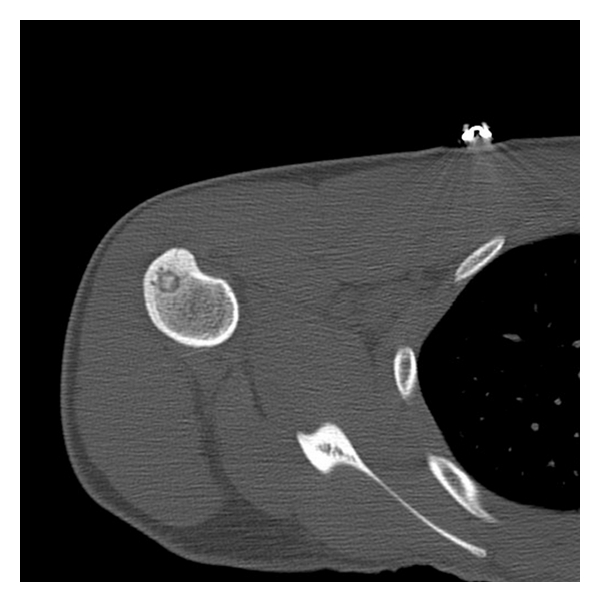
A Typical CT radiographic appearance of an osteoid osteoma in the proximal Humerus.
